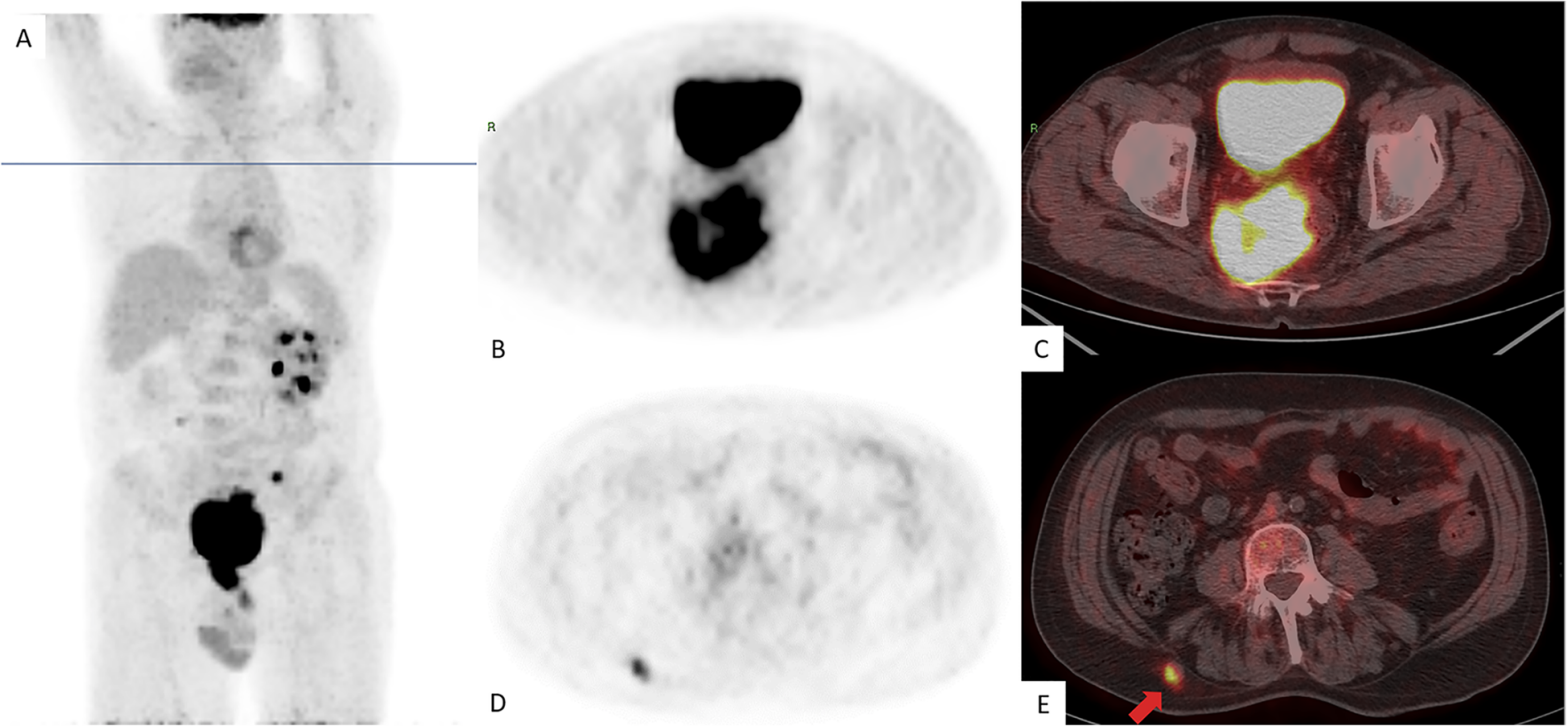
18F-FDG PET showing an extended area of disomogeneous abnormal hypermetabolism at the level of mass of the rectum, with both endoluminal and extraluminal expansion. Coronal plain (a), axial plain (b) and axial PET/CT (c). Pathologic hypermethabolism was confirmed at the level of the subcutaneous nodule of the right lumbar region (d, axial PET. e, axial PET/CT)
